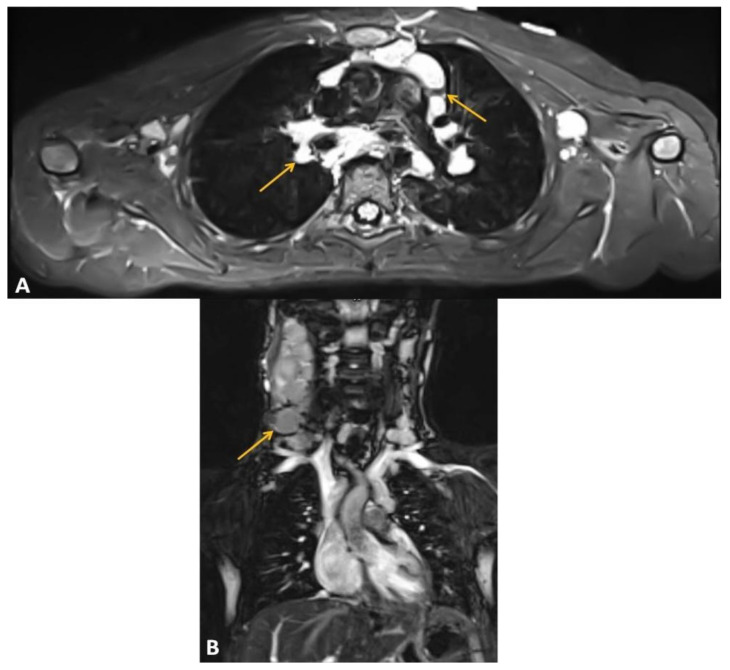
Chest MRI at the moment of LBCL diagnosis with the indication of carotic pathologic lymph nodes masses with arrows. (A) T2 STIR sequence axial. (B) T2 STIR sequence coronal.
